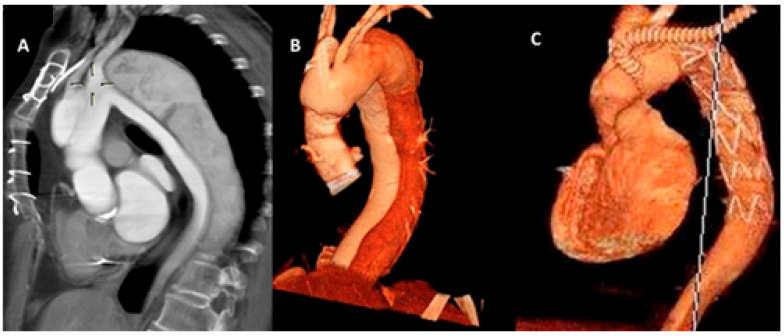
Preoperative (A,B) and postoperative (C) CT scan images showing chronic dissection involving the aortic arch and descending aorta with prominent false lumen. The distal hybrid graft anastomosis was performed in zone 2, with implantation of two supra-aortic vessels using the island technique and an extra-anatomic left subclavian artery (LSA) bypass.
